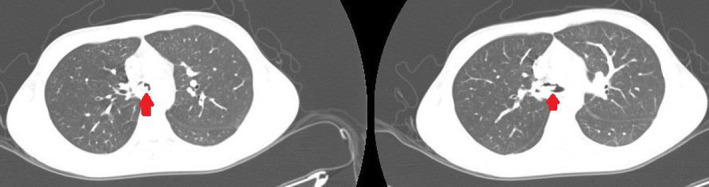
A computed tomography image of the second patient at the distal trachea and carina depicts severe tracheal narrowing and obstructing fungal mass in the right main bronchus (red arrow)
